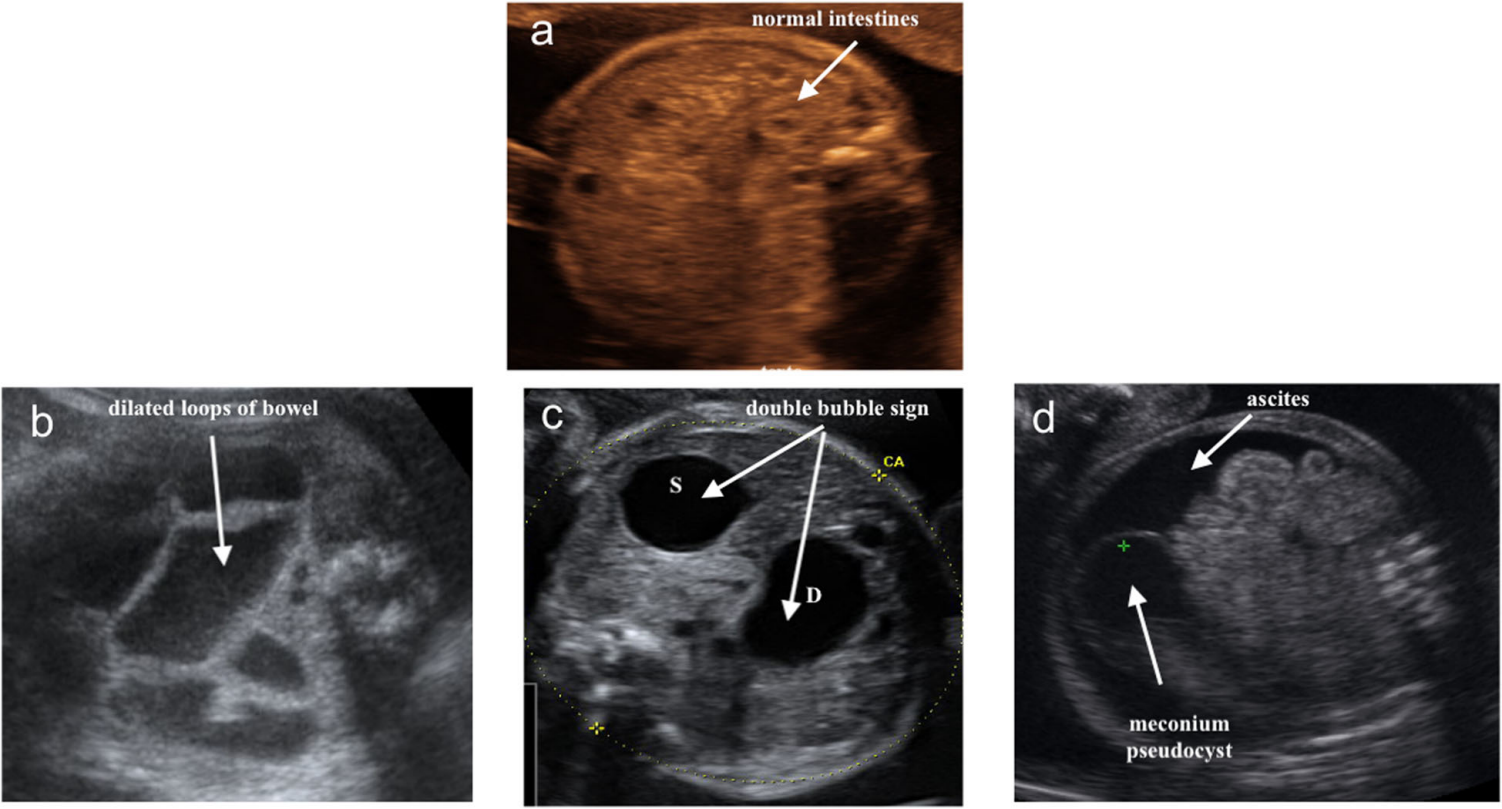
Ultrasound images comparing normal intestines and different signs of fetal bowel obstruction. a Normal intestines at 26 weeks of gestation, *white arrows*. b Ileal atresia in a fetus with gastroschisis at 30 weeks of gestation. c Double bubble sign (*white arrows*; S, stomach; D, duodenum) in a fetus with duodenal atresia at 28 weeks of gestation. d*White arrows*, ascites and meconium pseudocyst at 22 weeks of gestation
